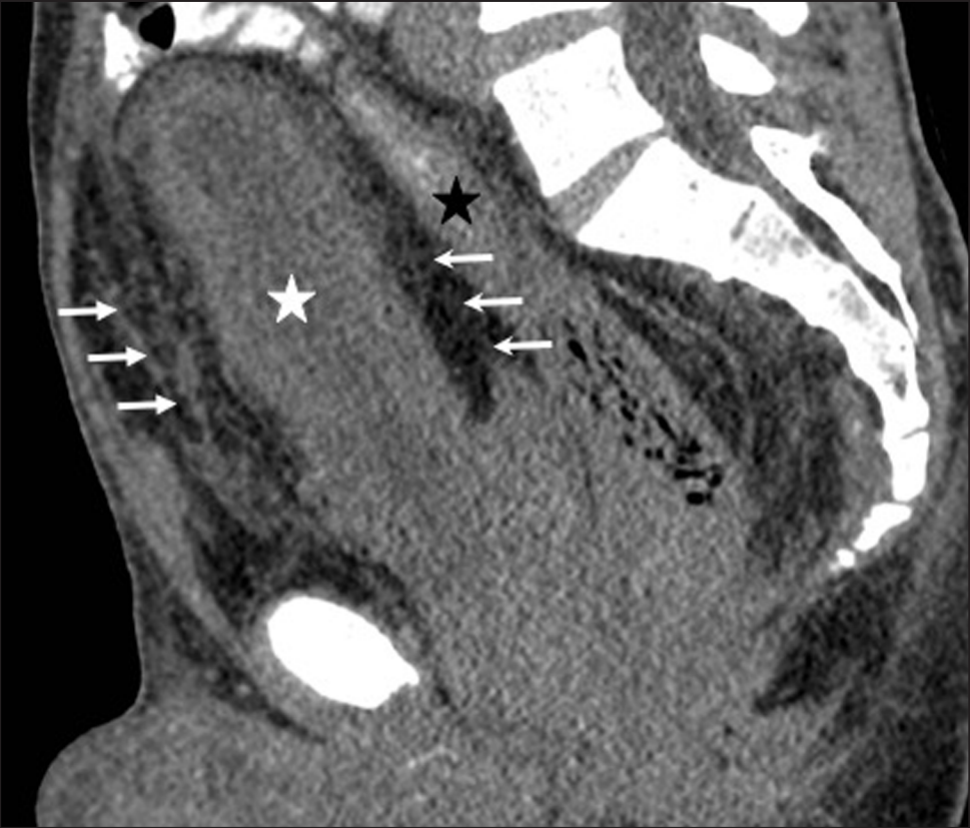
Contrast enhanced computed tomography. Sagittal image displaying the anatomical deformity of the bladder (white star) and the rectum (black star) caused by the deposition of hypodense fatty tissue (arrows).
